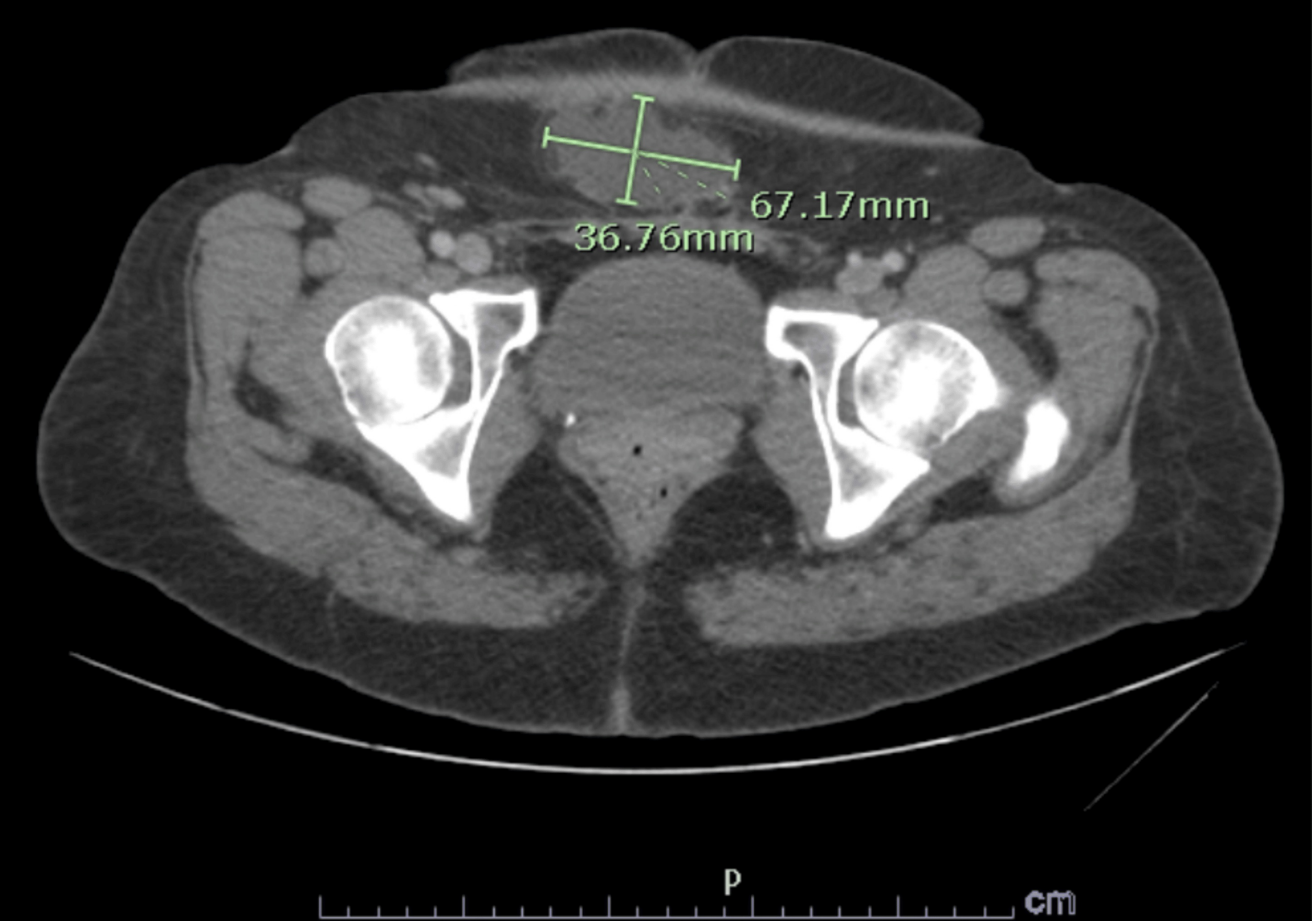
Axial CT abdomen view of the enlarging cystic mass that is now 6.7cm x 3.6cm. The nodule has irregular margins with mild spiculations and is directly adherent to the fibrotic, Pfannenstiel scar tissue.
